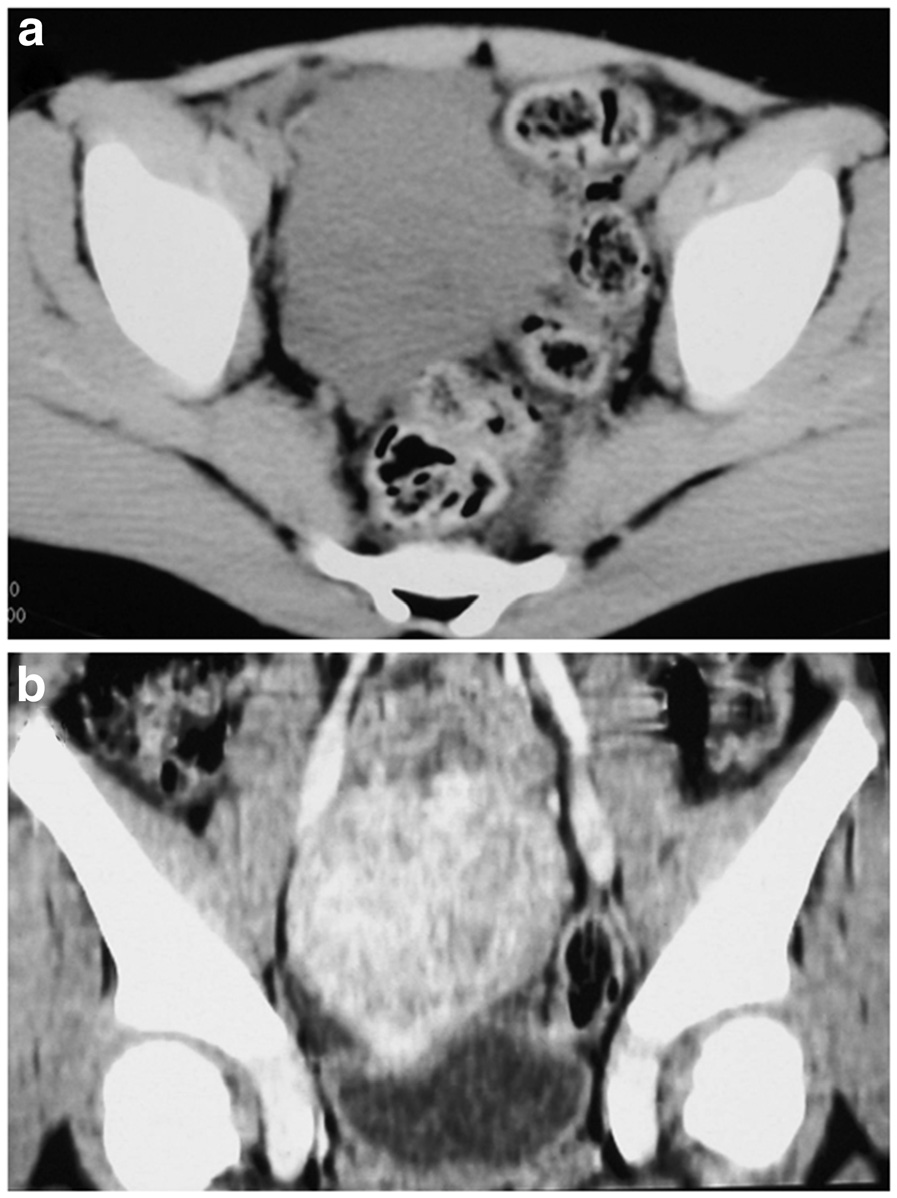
CT of the pelvis (a): Axial pre-contrast image shows a well-defined, slightly lobulated homogeneous soft-tissue mass in the right lower abdominal cavity. (b): Reconstructed coronal image shows the tumor with homogeneous enhancement without necrotic or cystic components.
